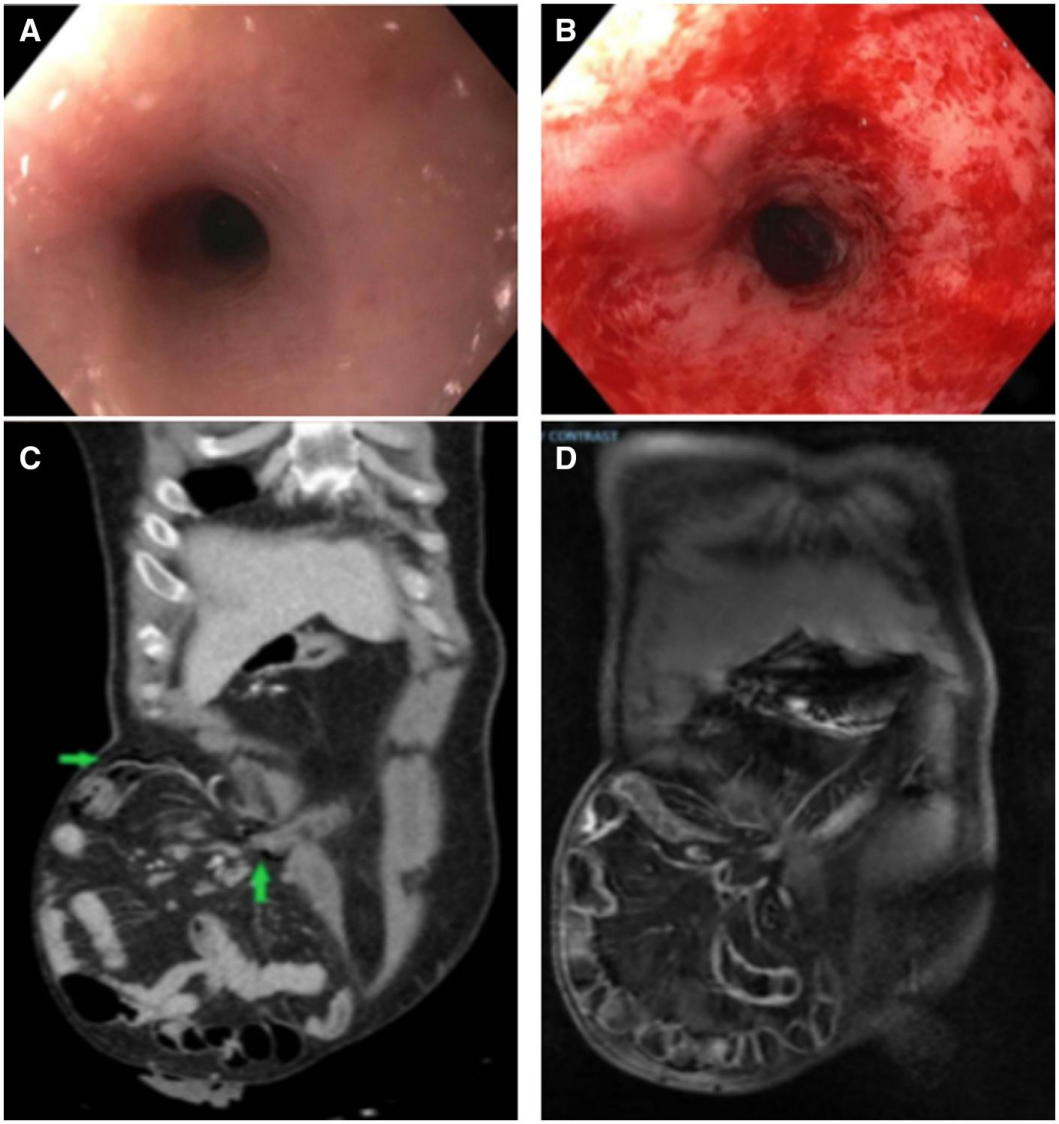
Endoscopic and imaging views of diversion colitis with air leak. (A) Mild anorectal ring stricture, traversable to a gastroscope. (B) Diversion colitis with friable mucosa. (C) Computed tomography following the ileoscopy and flexible sigmoidoscopy shows air leaks in the abdomen, as indicated by the arrows. (D) Magnetic resonance imaging after 1 week shows that previously seen air leaks in the abdomen have dissipated.
